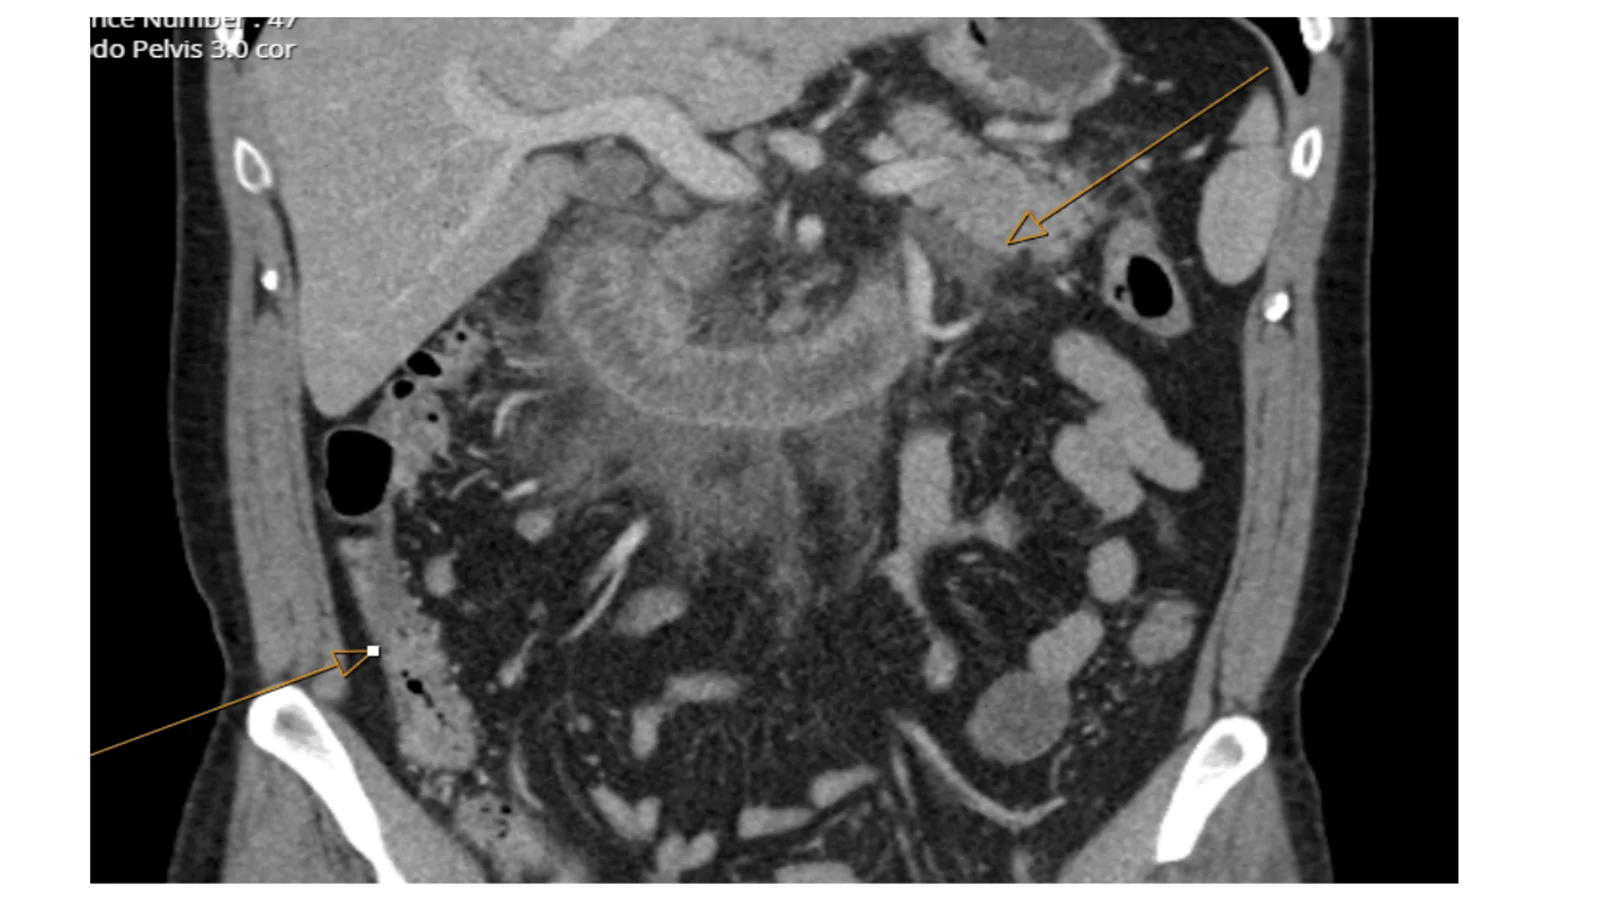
Coronal contrast-enhanced CT of the abdomen and pelvis showing normal wall thickness of the ascending colon (lower arrow) and features of acute pancreatitis with adjacent inflammation of the duodenum (upper arrow)
